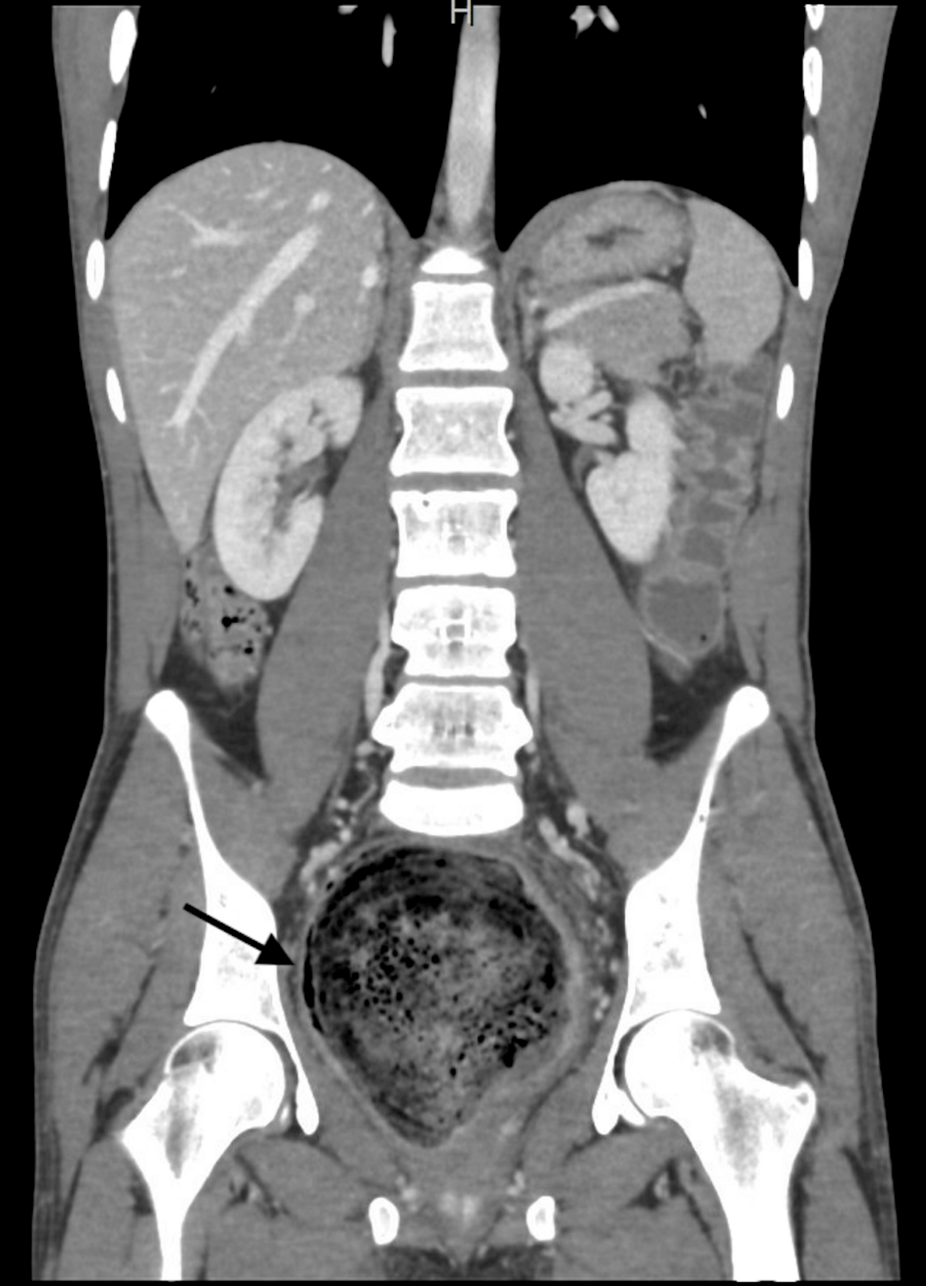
Coronal CT scan with contrast showing significant focal dilatation of the rectum with a large fecaloma inside, measuring about 12.2 cm in length, 8 cm in width, and 10 cm in height. This is an image of a huge dilated rectum that is loaded with feces.
